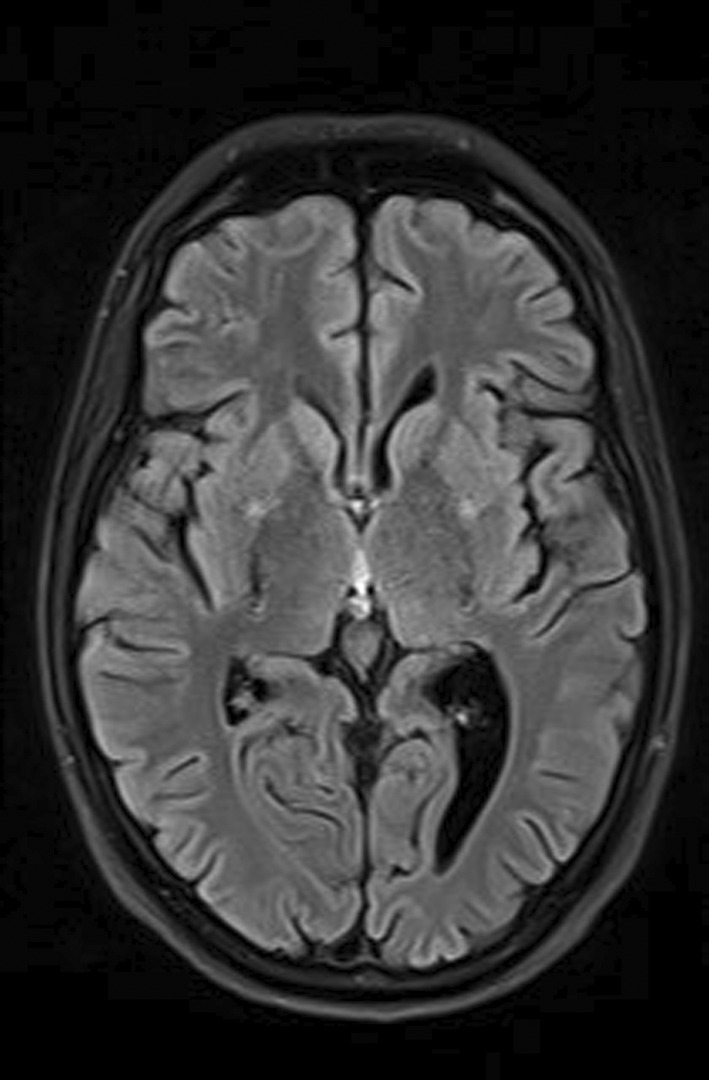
Magnetic resonance imaging (MRI) and magnetic resonance angiogram (MRA) of the brain.
